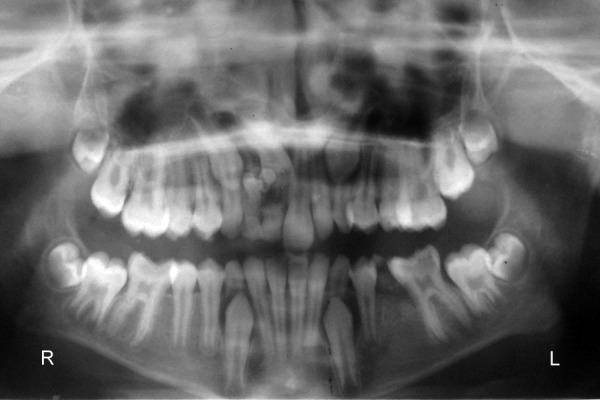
Panoramic radiograph showing a single calcified mass
